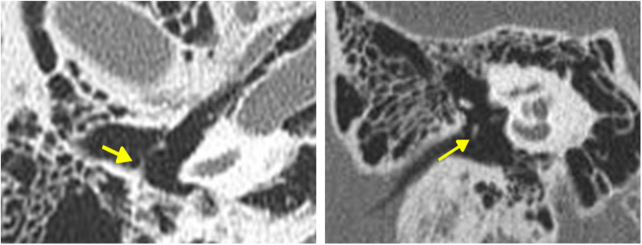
The congenital malleus bar (arrows) in axial (right) and coronal (left) view of temporal bone CT.
